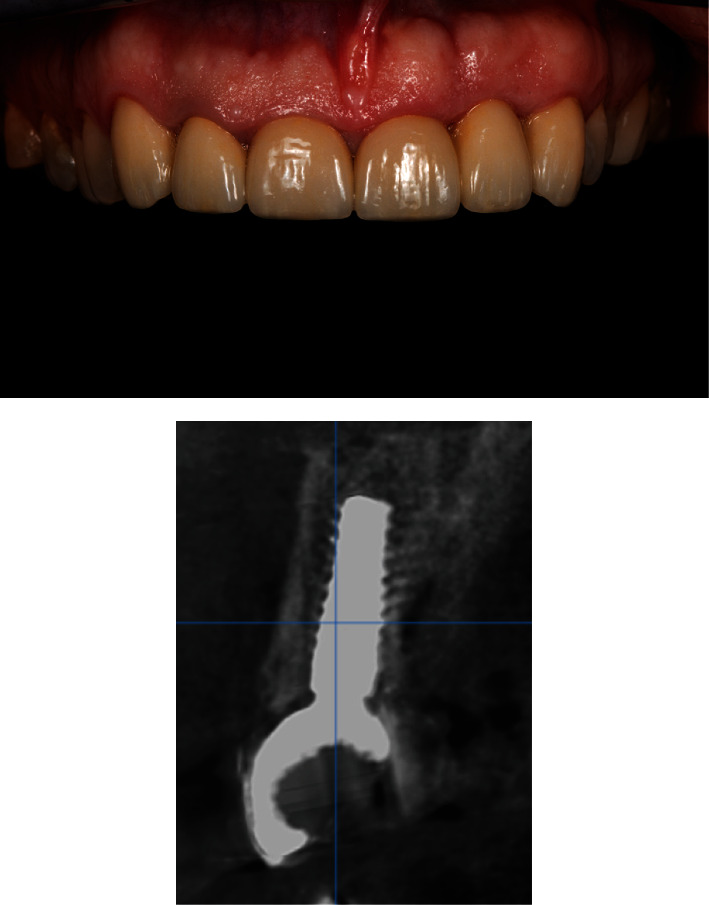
The final restoration in place with control CBCT after 2 years post-operative.
